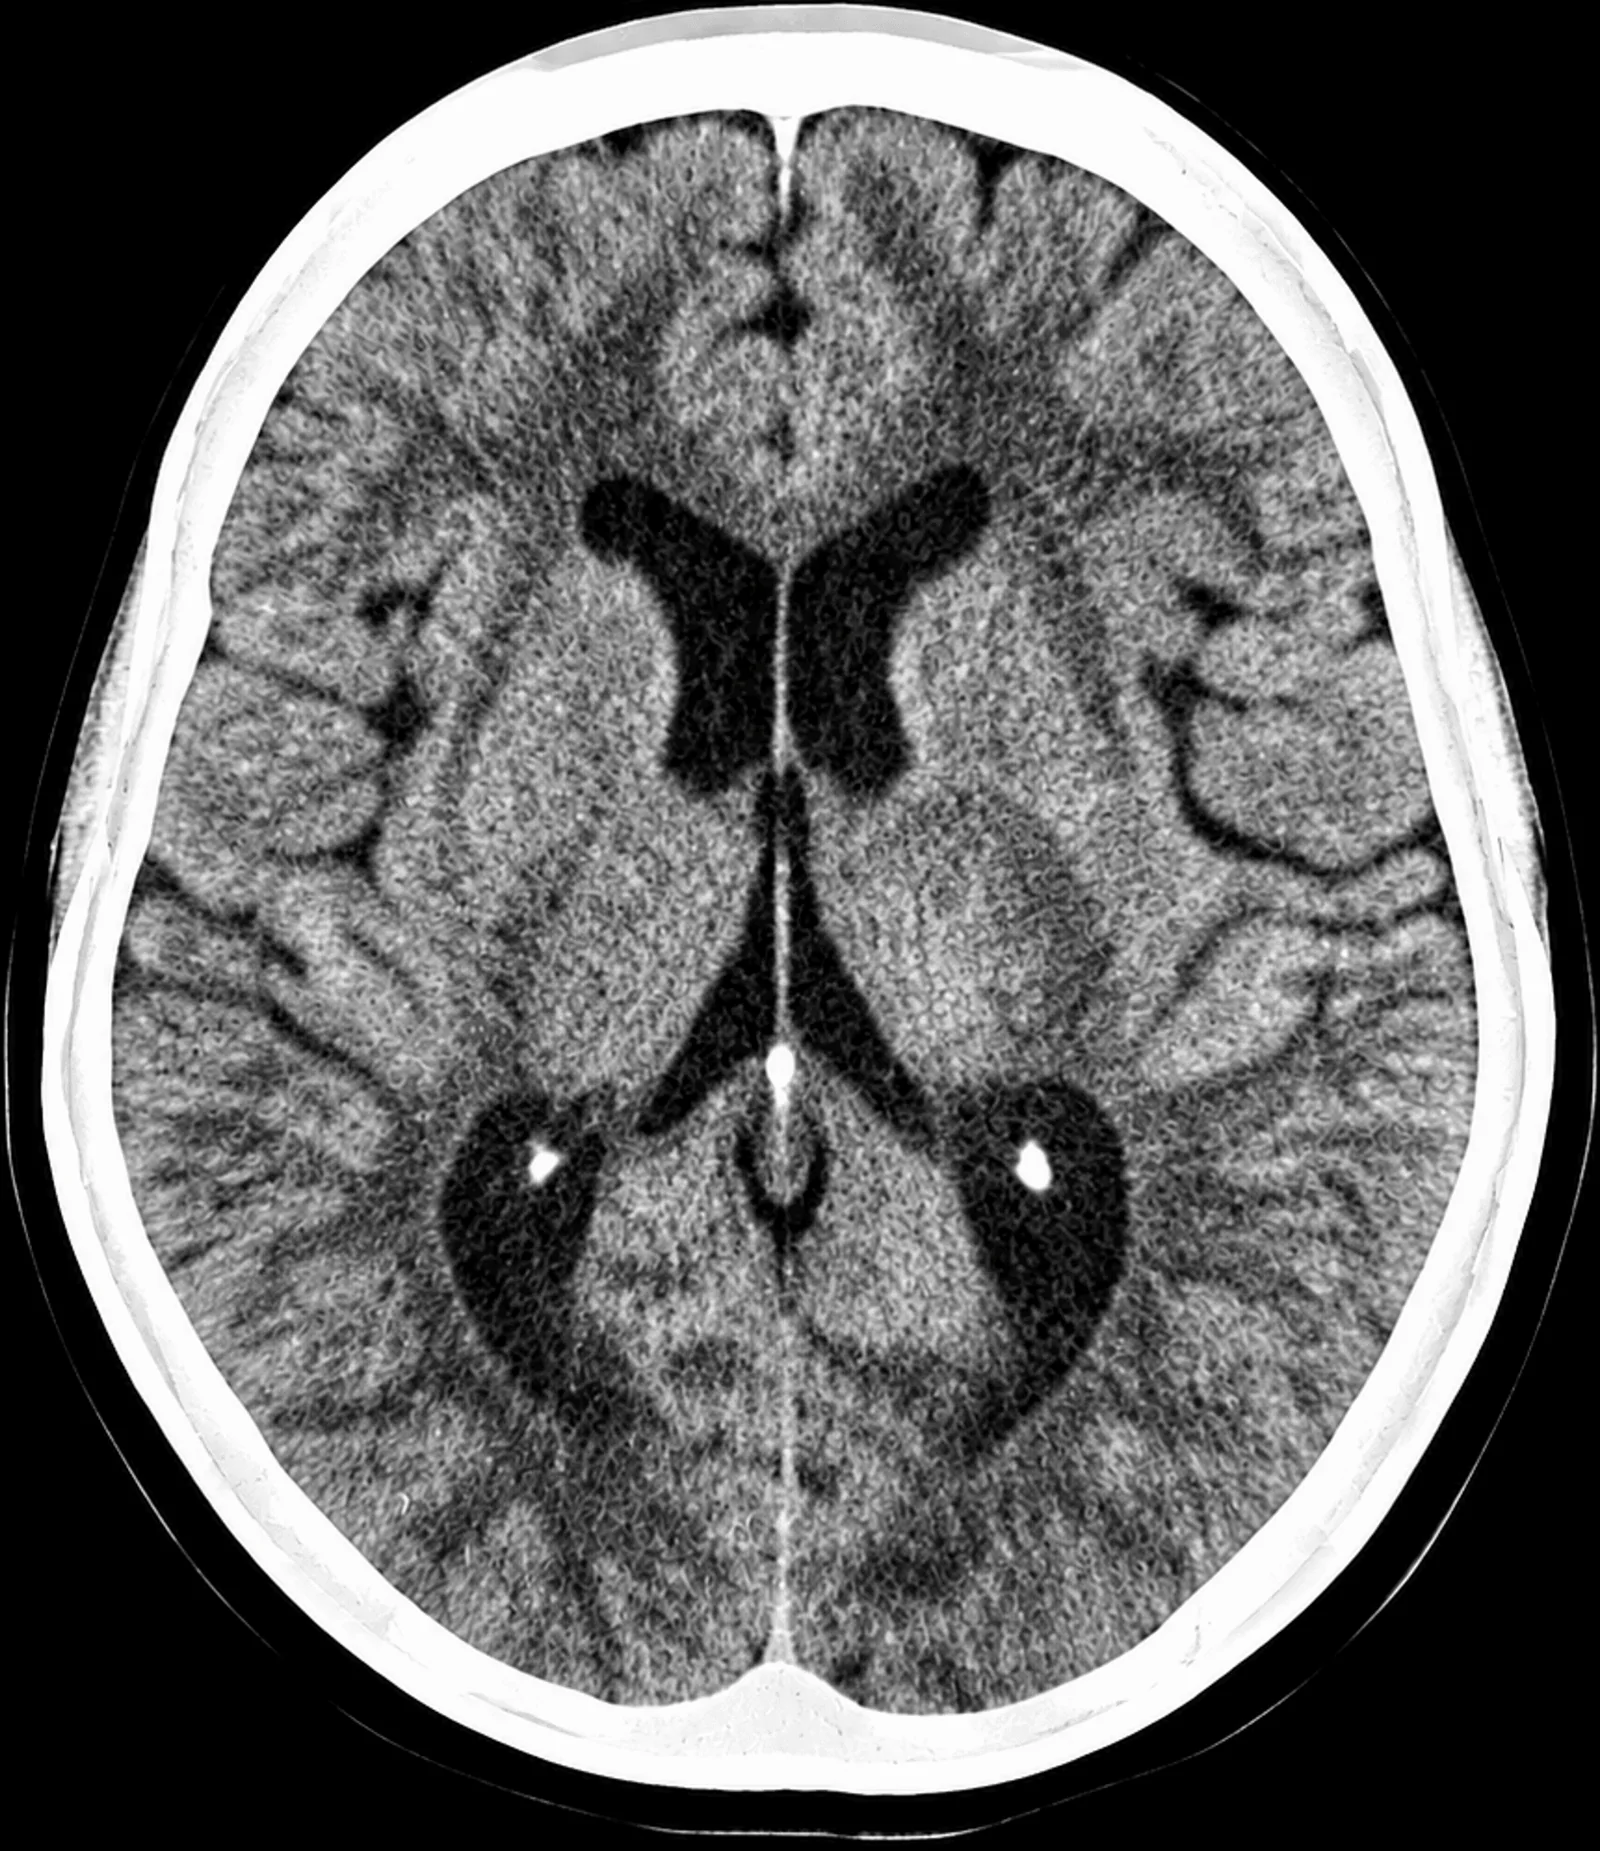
Normal CT brain when the patient developed myoclonus
